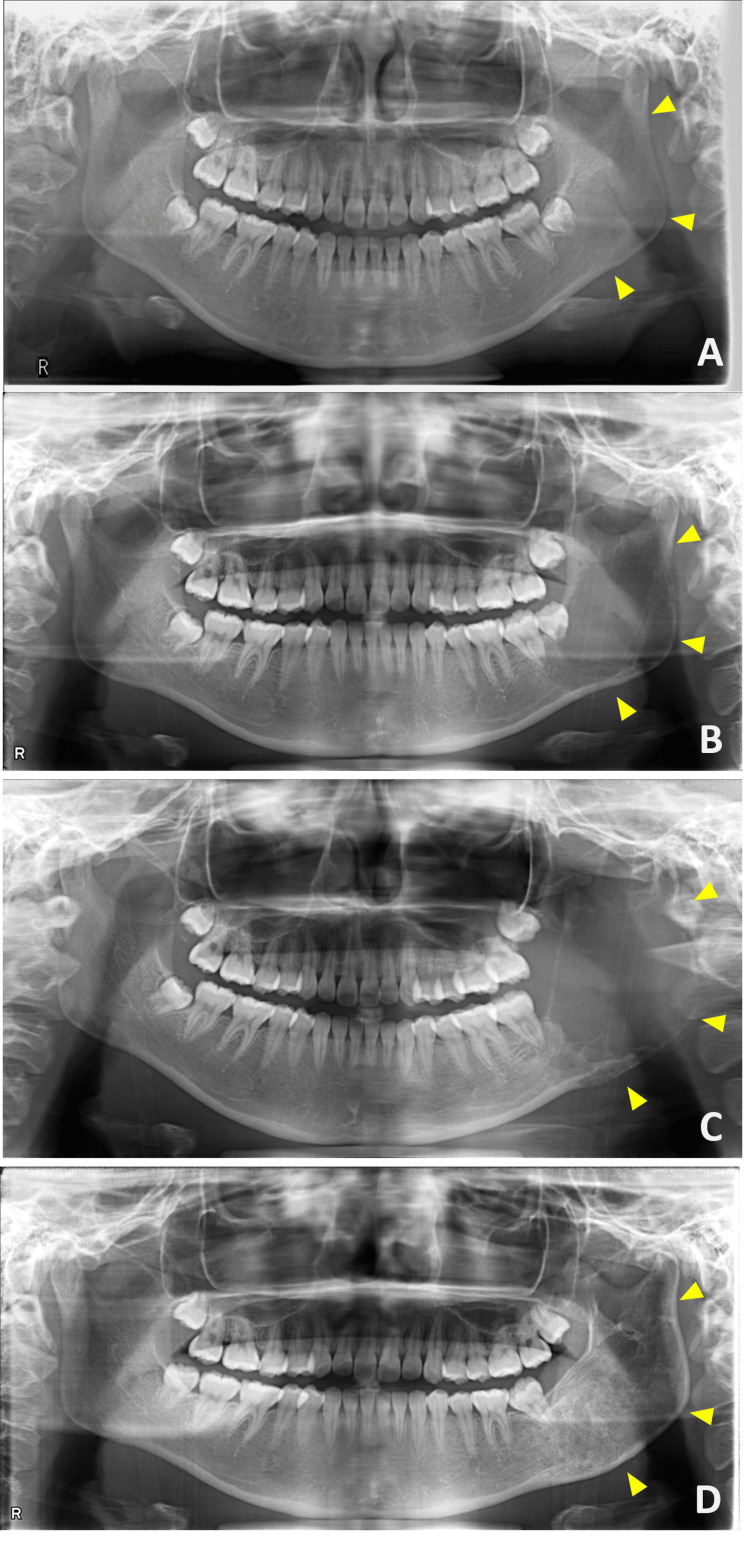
Orthopantomogram X-ray (A) Eight months prior to the initial visit, no abnormalities were observed. (B) At the initial visit, a radiolucent area was observed in the left mandibular ramus. (C) Before sclerotherapy, the radiolucent area had increased in size. (D) At 24 months post operation, the lesion had resolved, with evidence of bone regeneration. The arrows indicate the mandibular ramus before and after the onset of the lesion, as well as after healing.
